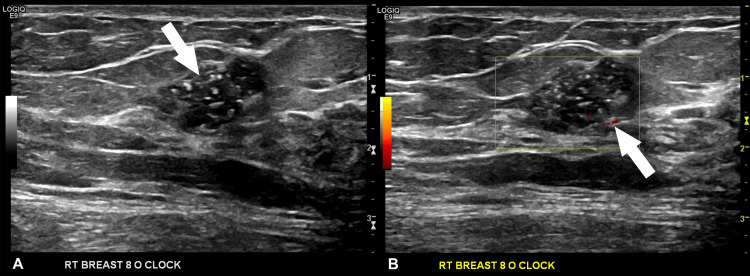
(A) Ultrasound shows an 18 x 10 mm solid mass at the 8 o’clock position of the right breast; (B) Internal calcifications are seen, and Doppler analysis shows vascularity with a small amount of blood flow in the periphery
